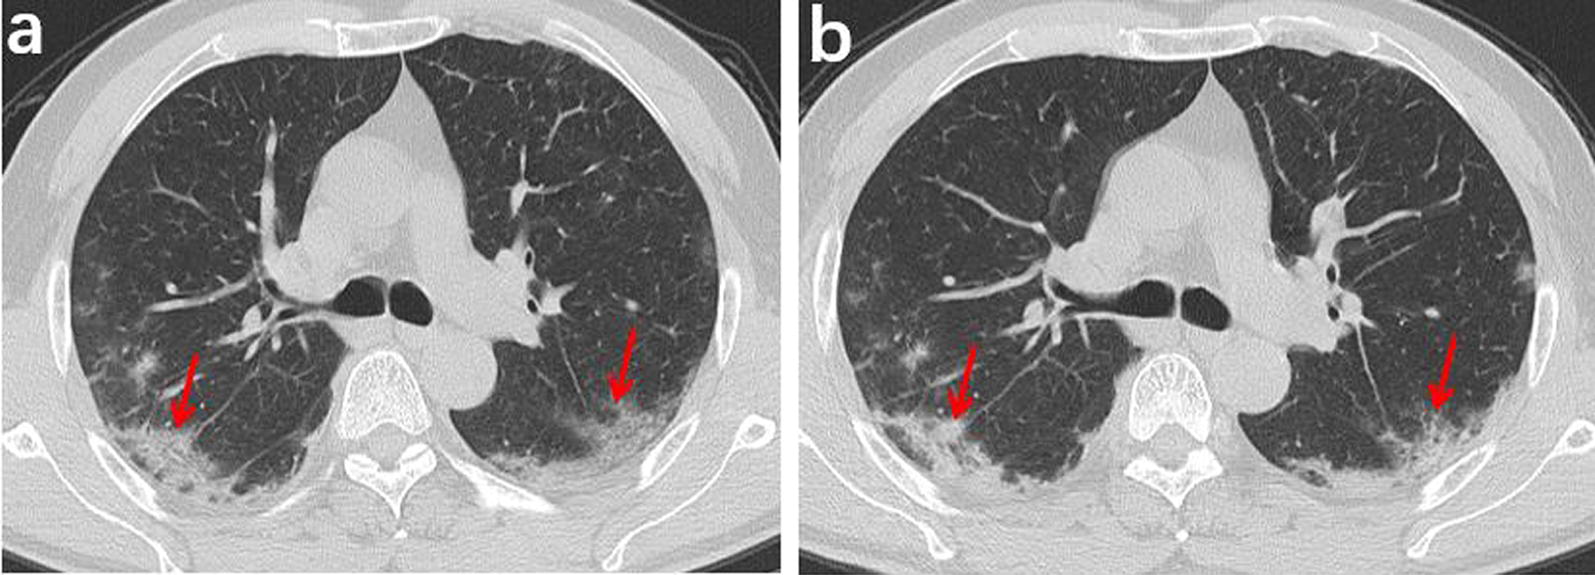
A 55-year-old male who had close contact with people in Wuhan presented fever for 8 day. a The initial axial chest CT shows mixed shadows of ground-glass opacities and consolidations with peripherally distributed (red arrows). b The follow-up axial-view chest CT shows no significant changes after 4 days later (red arrows)
